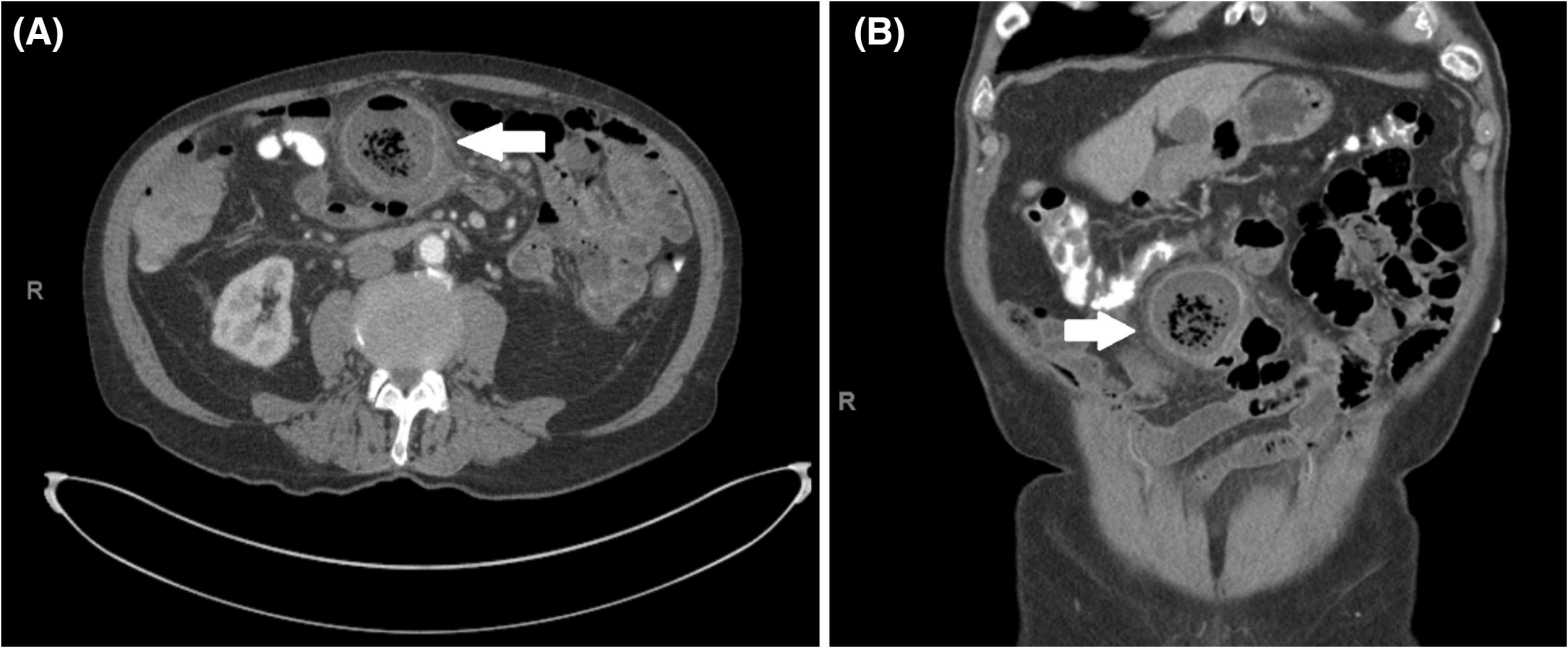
Contrast‐enhanced CT scan of the abdomen/pelvis: (A) axial image. (B) Coronal image. Prominent small bowel diverticulum measuring 5.2 × 5.4 cm with internal fecal contents, with tall thickening and adjacent fat stranding (arrow)
